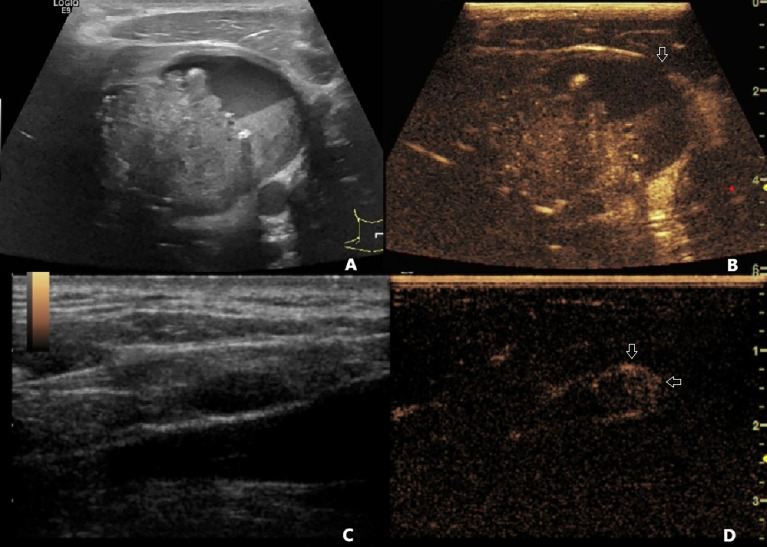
(A) A 40-year-old male patient with pathology-confirmed PTC underwent preoperative US and CEUS examination. (A) One 6.8 cm * 4.7 cm mixed cystic and solid nodule was found in the left lobe of the thyroid. The contact range between PTC and the thyroid capsule was >50%. The capsule was continued on US. (B) On CEUS, the contact range between PTC and the thyroid capsule was >50%, and discontinued capsule was observed (white arrow). (C) A 1.2 cm * 0.5 cm hypoechoic lymph node with clear boundary and unclear hilum structure was found on US. (D) This lymph node demonstrated centripetal perfusion and peripheral enhancement on CEUS (white arrows). This patient was diagnosed with non-invasive PTC by US, PTC with ECE and LNM by CEUS and US+CEUS, and was finally confirmed to have PTC with ECE and LNM by pathology. PTC, papillary thyroid carcinoma; US, ultrasound; CEUS, contrast-enhanced ultrasound; ECE, extracapsular extension; LNM, lymph node metastasis.
